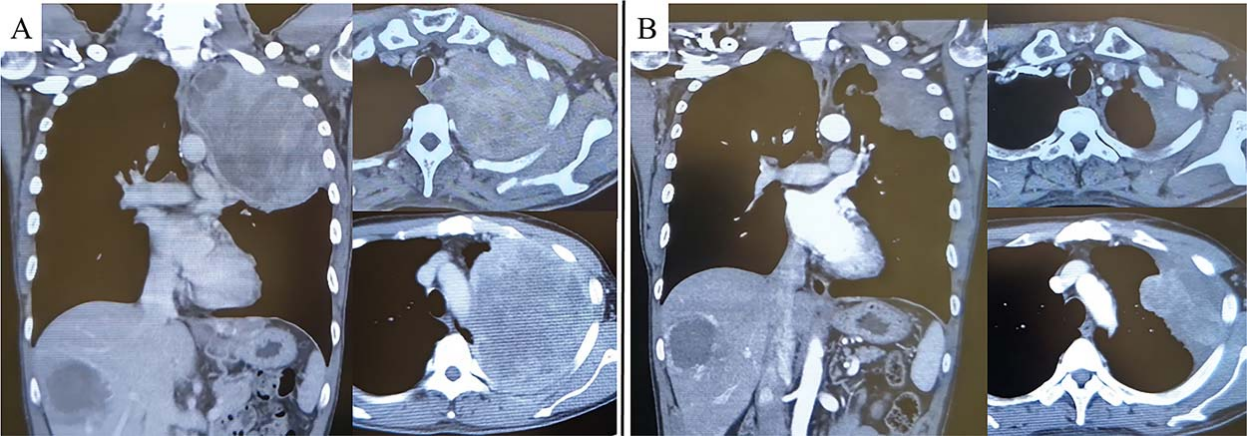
Pre-treatment CT revealed a large mass exceeding 13 cm in the left lung apex, with invasion into the first through fifth ribs (A). Following treatment with nivolumab with ipilimumab, the tumor showed marked shrinkage (B).
